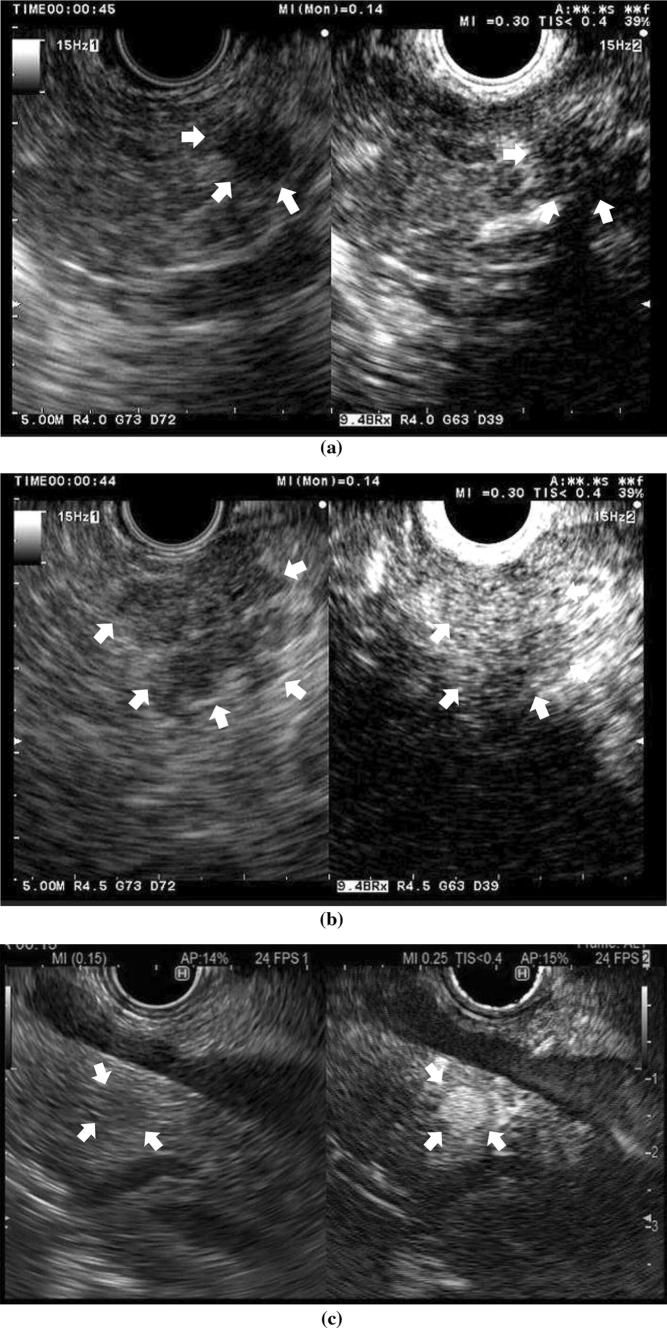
a A typical example of a solid lesion with hypoenhancement (a ductal carcinoma of 10 mm). Conventional EUS shows a hypoechoic area (arrowheads) at the pancreas body (left). Contrast-enhanced harmonic endoscopic ultrasonography (CH-EUS) indicates that the area is hypovascular (arrowheads) compared with the surrounding tissue (right). b A typical example of a solid lesion with isoenhancement (Autoimmune pancreatitis). Conventional EUS shows a hypoechoic area (arrowheads) at the pancreas head (left). CH-EUS indicates enhancement in this area similar to the surrounding tissue (arrowheads) (right). c A typical example of a solid lesion with hyperenhancement (a neuroendocrine tumor of 8 mm). Conventional EUS shows a hypoechoic mass (arrowheads) at the pancreas head (left). CH-EUS indicates that enhancement in the mass is higher than in the surrounding tissue (arrowheads) (right)
